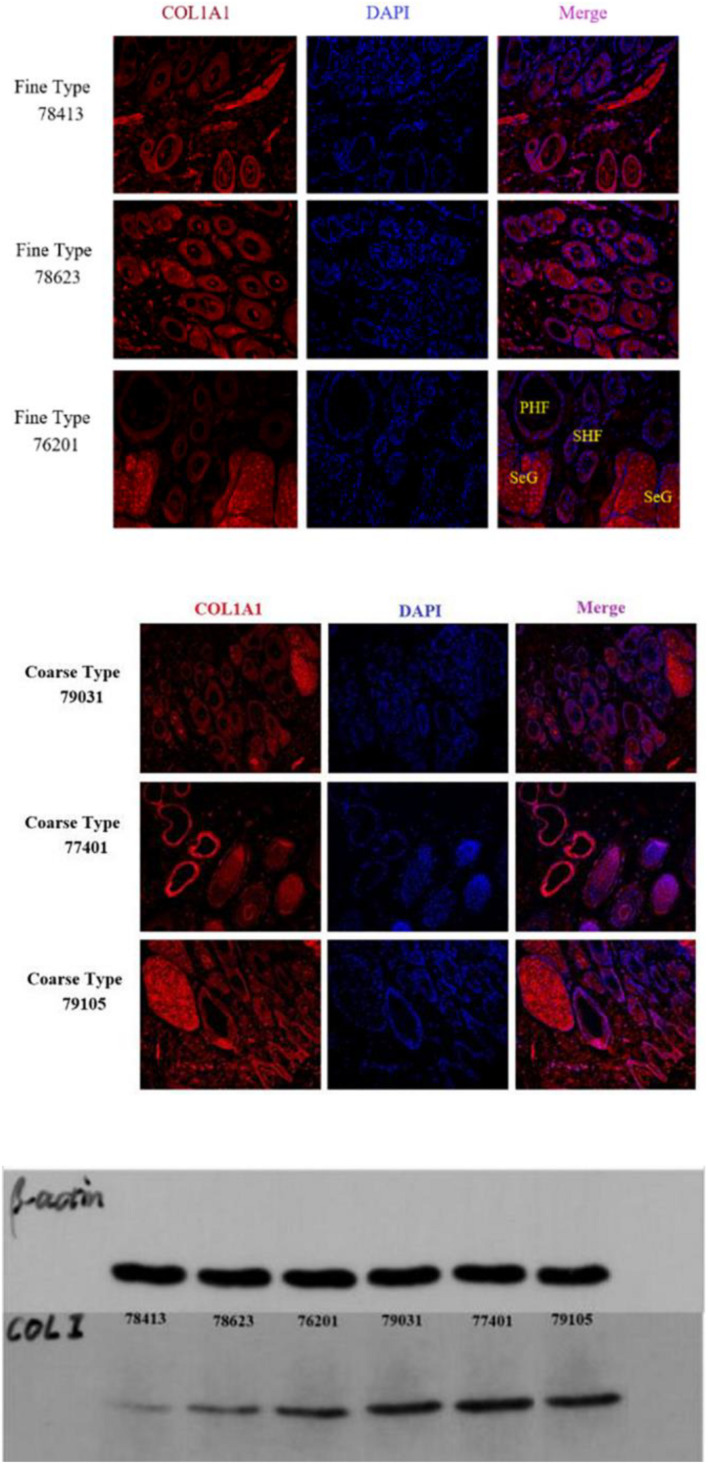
IF results of *COL1A1* in FT-LCG and CT-LCG skin tissues (200x).
